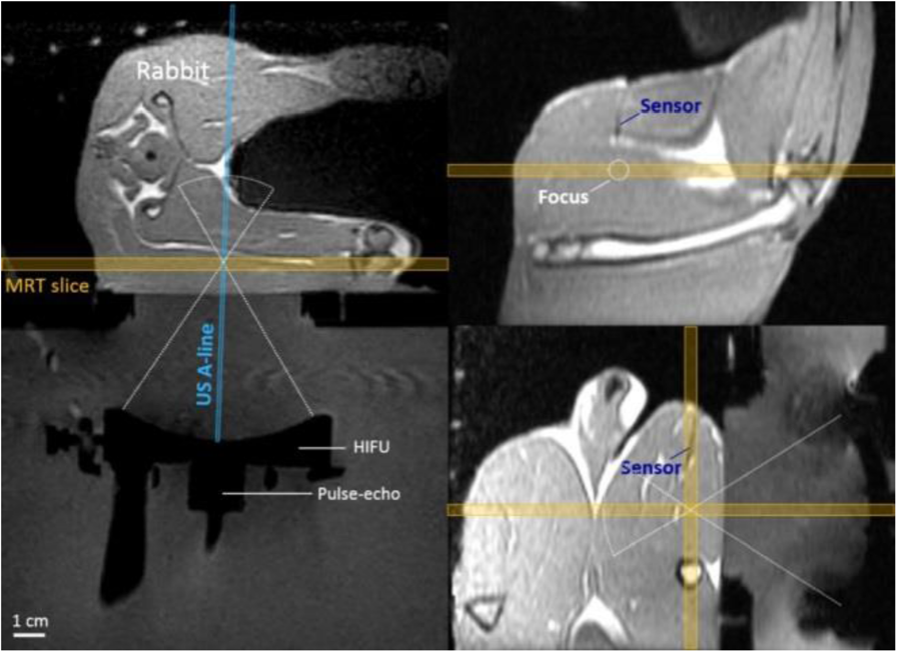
3D T1-weighted MRI of *in vivo* experiment setup for simultaneous ultrasound and MR thermometry in rabbits
